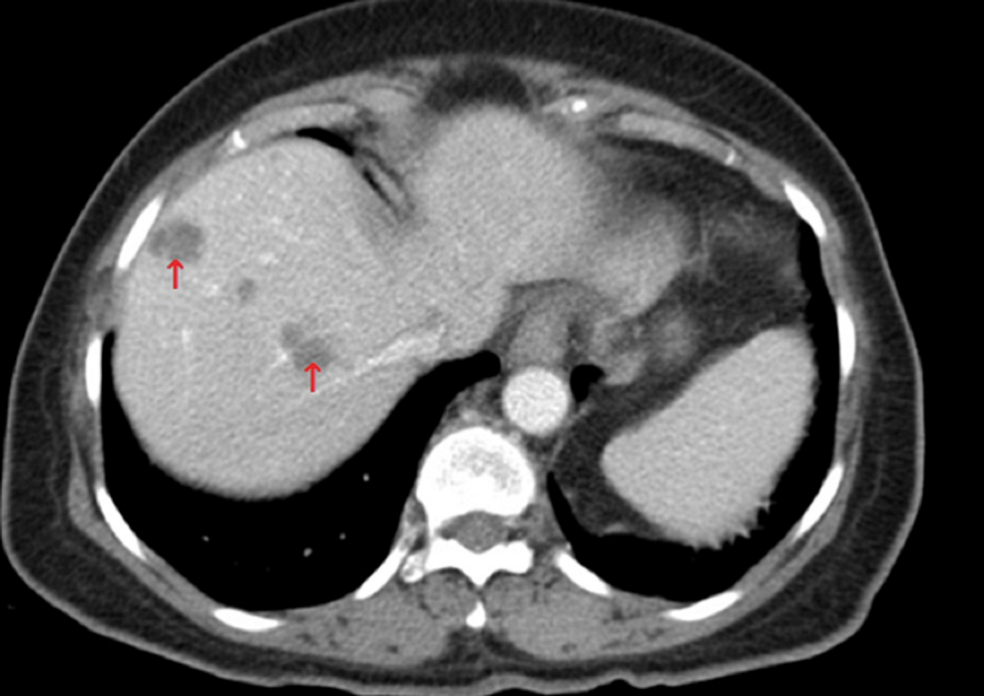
CT A/P axial view demonstrating multiple, hypoattenuating, ill-defined cystic lesions in the liver (red arrows) with peripheral enhancement and internal septation CT A/P: computed tomography of the abdomen and pelvis
